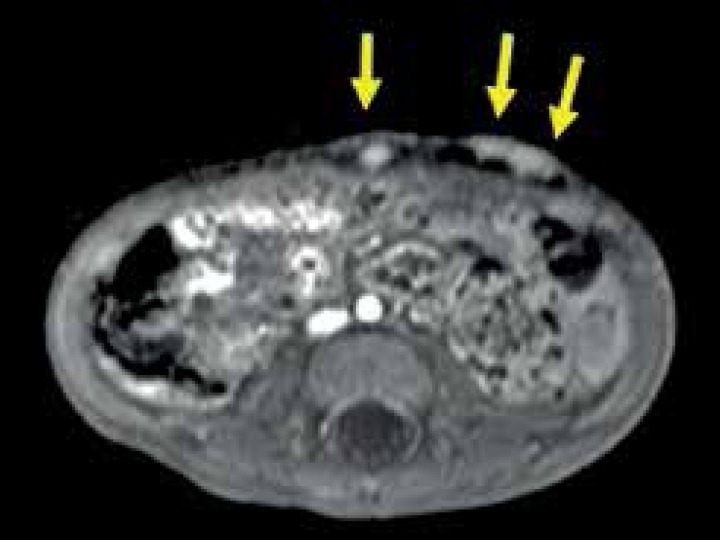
MRI of the abdomen. Arrows indicate multiple, subcutaneous nodules
